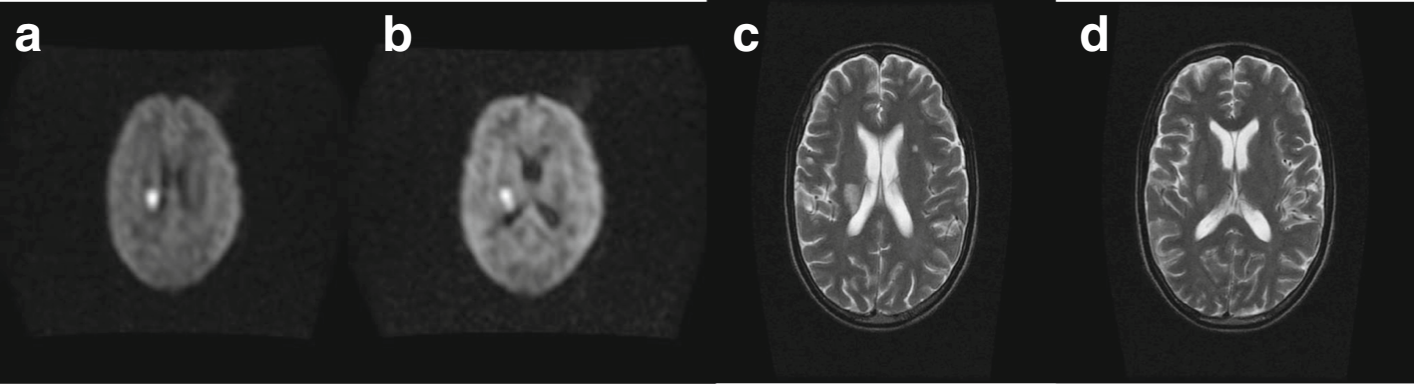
a-d are MRI sequences taken on day 5 of an acute presentation of left arm and leg weakness; a-b are DWI axial B-900 sequences, showing restriction in the right corona radiata (a) and posterior limb of the internal capsule (b). c and d (T2 axial sequences) showed high signal in the corresponding areas to the DWI lesions; consistent with an acute ischemic stroke. High T2 signal was also seen in the left deep white matter (c); Not shown but there was increased T2 signal (with DWI restricted diffusion) in the right cerebral peduncle and the brainstem (without DWI changes); suggesting further ischaemia
